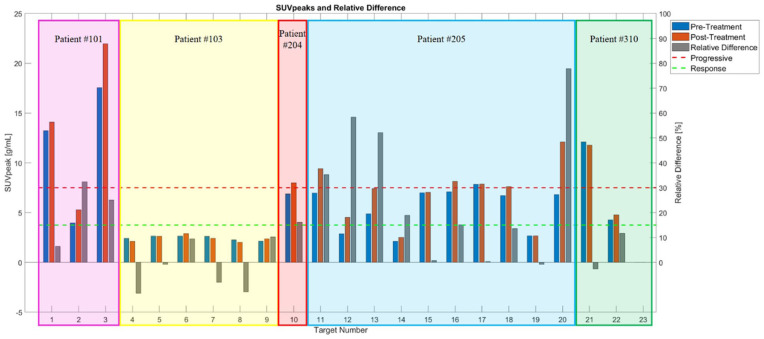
SUV_peak_ and RD for all 23 targets across subjects. SUV_peak_ values from pre- and post-treatment are shown in blue and orange bars, respectively, with the *y*-axis scale on the left. The RD, representing the percentage change in SUV_peak_ between pre- and post-treatment, is displayed in gray bars, with the corresponding *y*-axis on the right. The green dashed line marks the lower threshold, below which a target is considered responsive to our monotherapy. The red dashed line marks the upper threshold, above which a target is classified as progressive. Values of RD falling between these 2 dashed lines indicate changes that are not significant enough to categorize the target as either progressive or responsive, and therefore, categorized as indeterminate. RD, relative difference.
